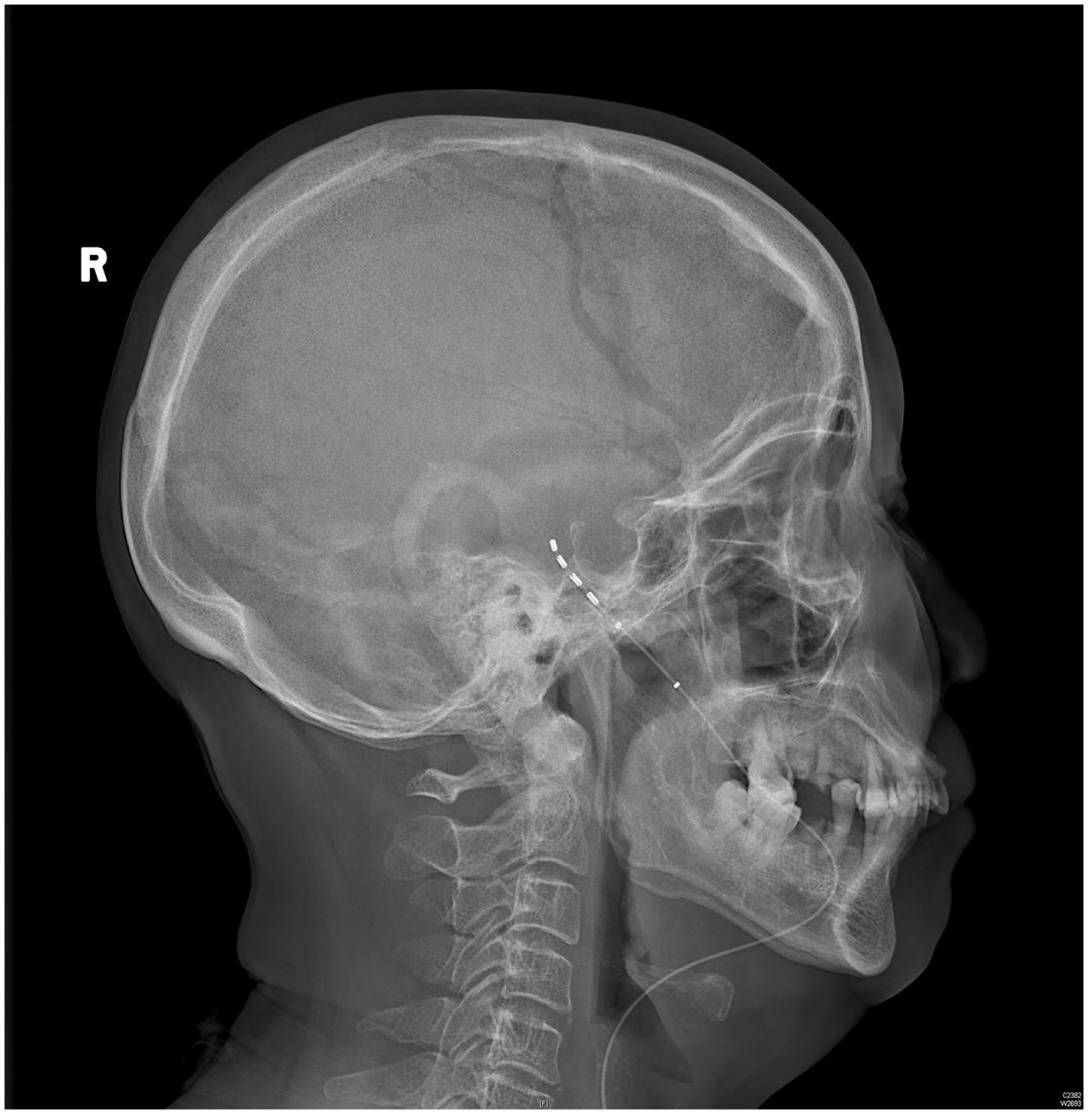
A skull-base x-ray of the lateral view.
